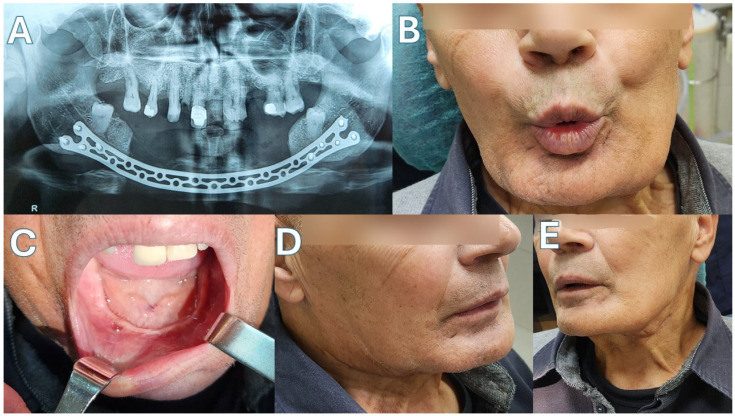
Case 1. (A) Post-op panoramic X-ray. (B) The patient 5 months after the operation, showing good marginal mandibular nerve function. (C) Intraoral view. (D) Right mandibular contour. (E) Left mandibular contour.
